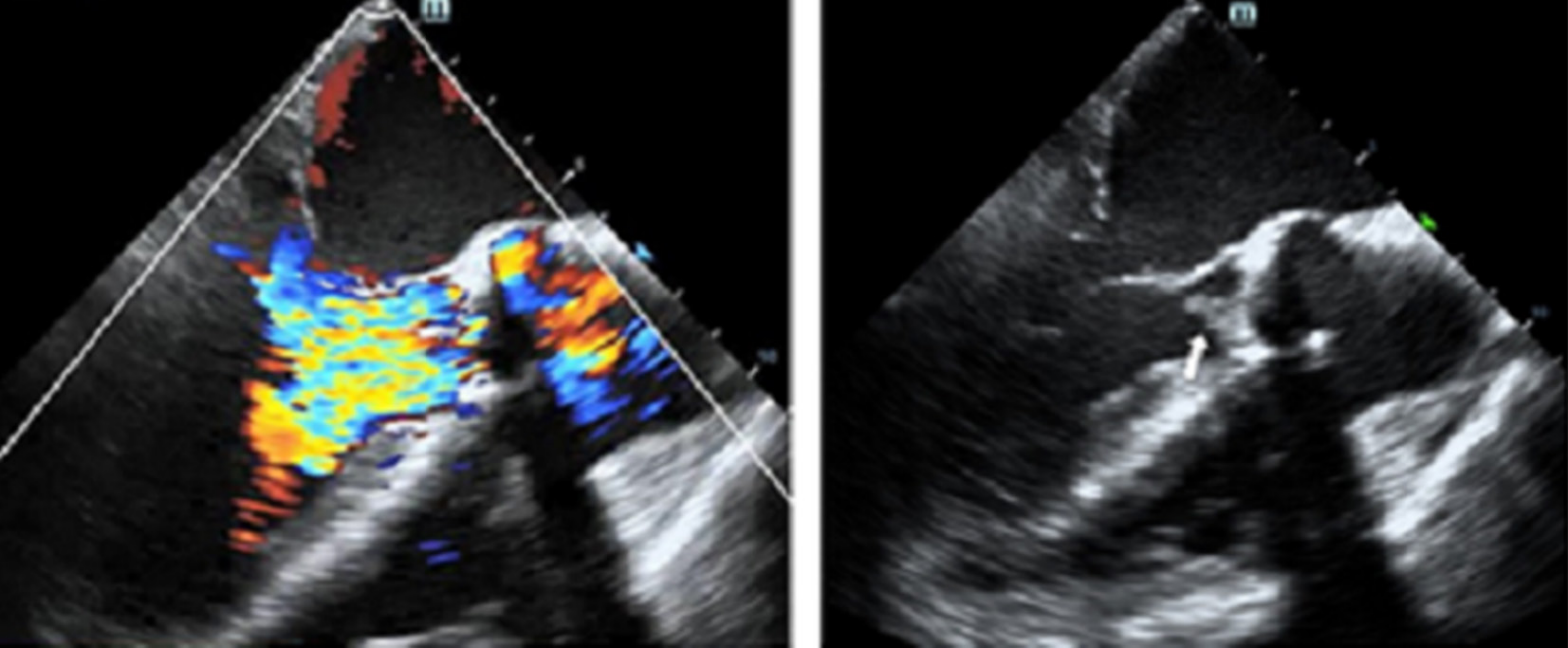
Transesophageal echocardiography before Redo‐TAVR.
